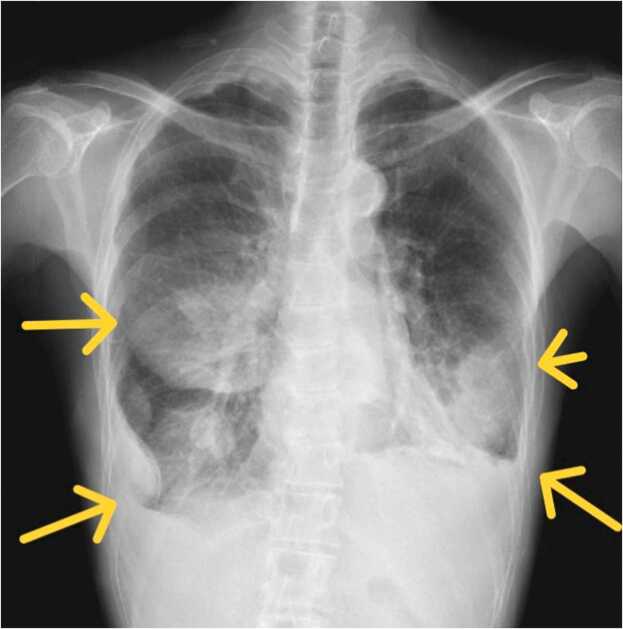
Chest radiograph on admission. That revealed the lungs were hypolucent bilaterally, with dullness of the costophrenic angle (yellow arrows).
